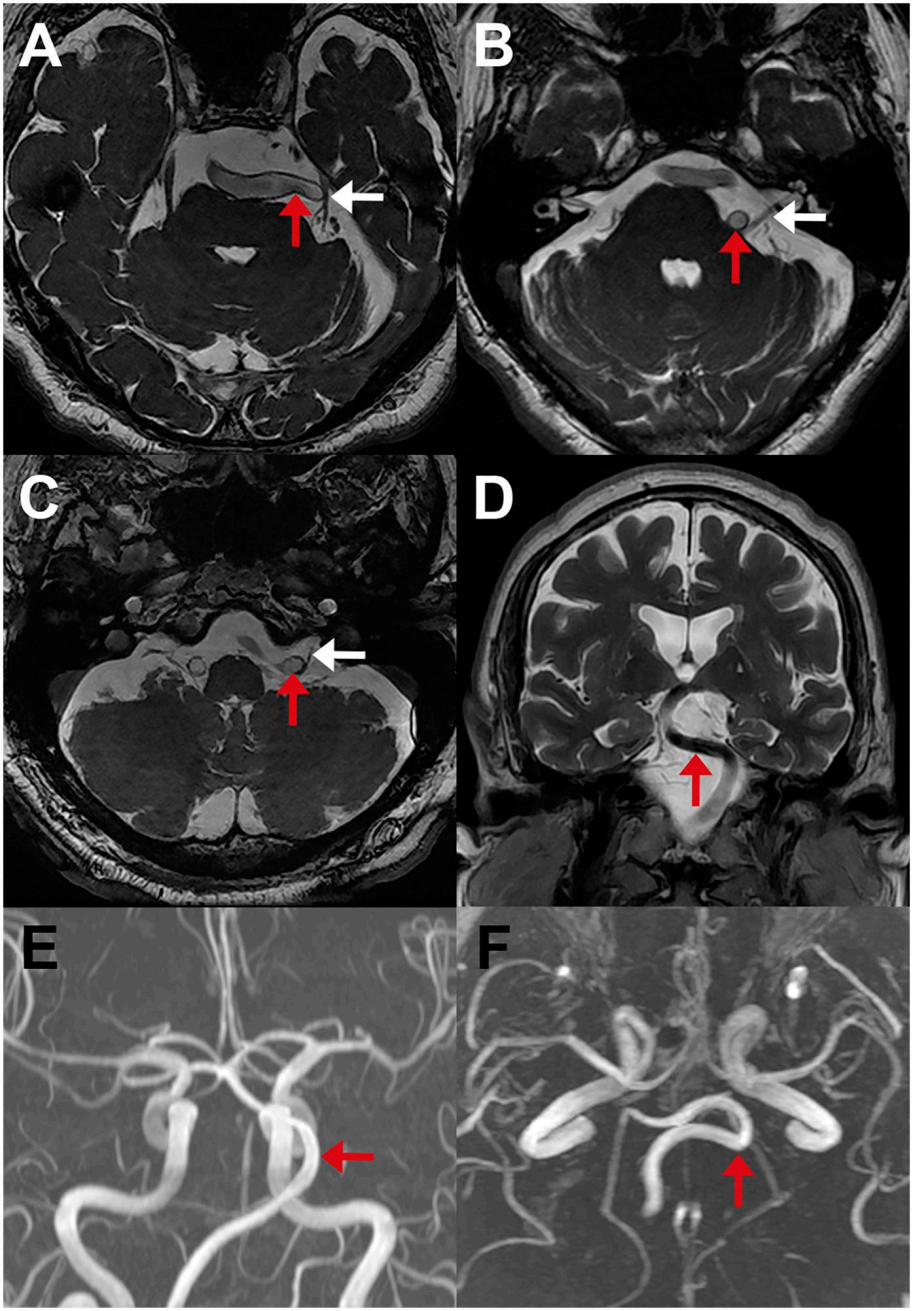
Preoperative neuroimaging findings in patients with cranial nerve compression due to VBD. (A–C) MRI 3D-FIESTA sequences showing compression of the CN V (A), CN VII (B), and CN IX (C) by the VBA (red arrows); corresponding nerves are indicated with white arrows. (D) Coronal MRI suggesting displacement of the brainstem by the VBA (red arrow). (E,F) Axial and coronal MR angiography demonstrating the tortuous course of the VBA (red arrows).
